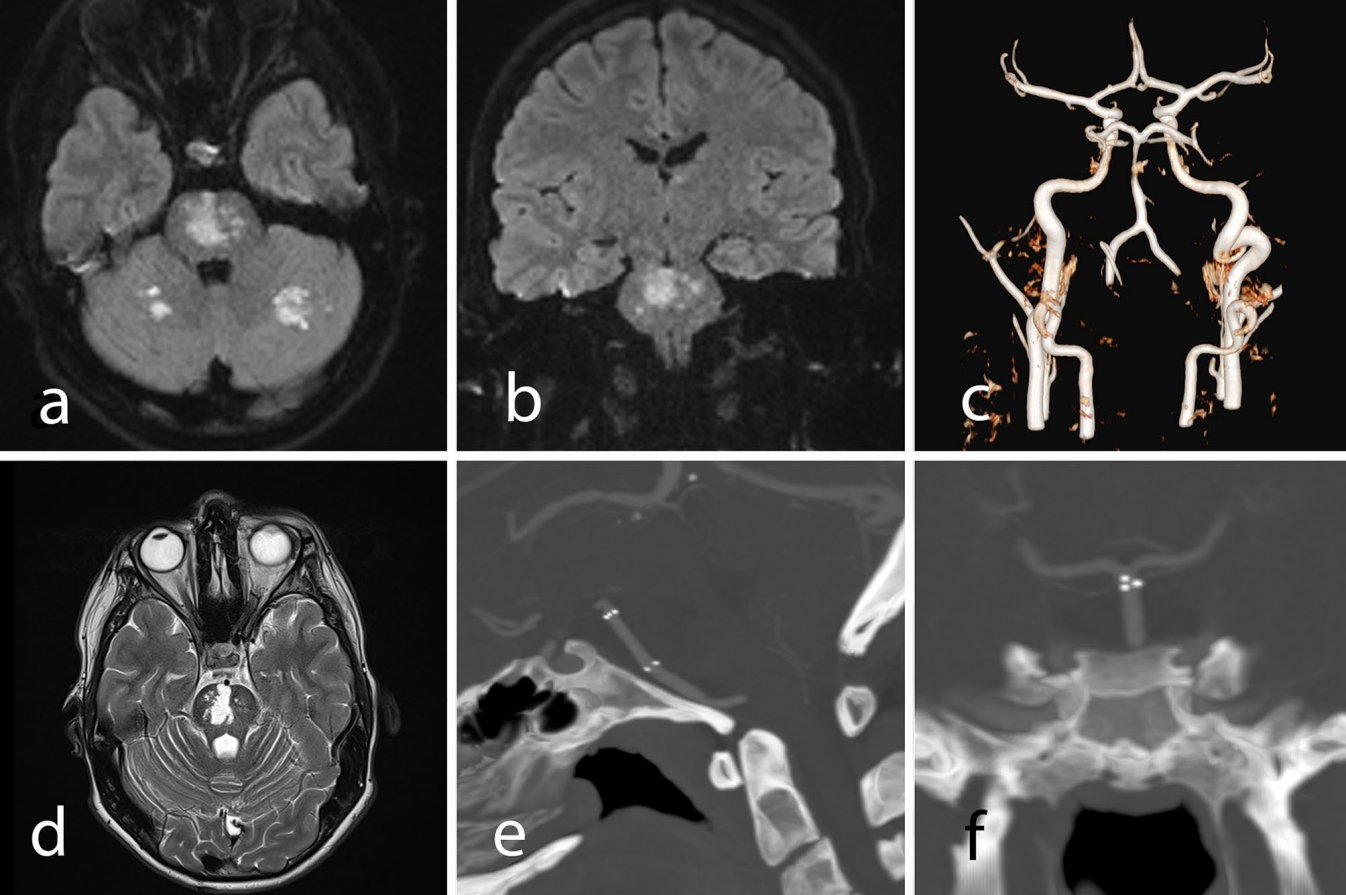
a and b Day 1 follow-up MRI: diffusion-weighted images show acute infarction involving the upper third of pons. Signs of fresh ischemia in both cerebellar hemispheres. c Day 1 follow-up MR angiography, 3D reconstruction, shows flow in basilar artery and its branches. The lumen is only seemingly narrowed—a consequence of the artifacts caused by a metal stent inserted into the basilar artery. d 1-month follow-up MRI shows post-ischemic, fluid-filled cavity occupying more than one-third of cross-section of the upper pons. e and f 10-month follow-up, CT angiography shows stent in basilar artery, reconstructed lumen of the basilar artery, and normal flow in all its branches
